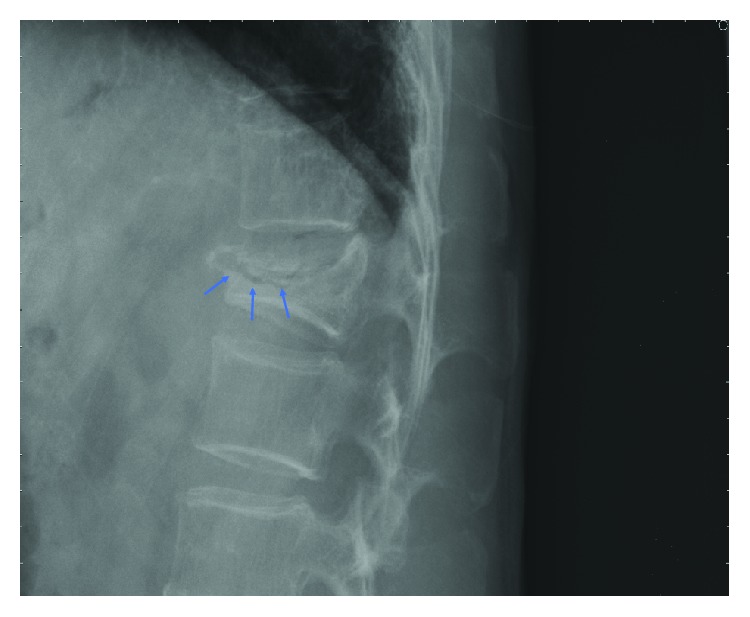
Lateral plain radiograph of the thoracolumbar spine. The arrows point to the crescent-shaped shadow in the vertebral body, that is, the IVC.
